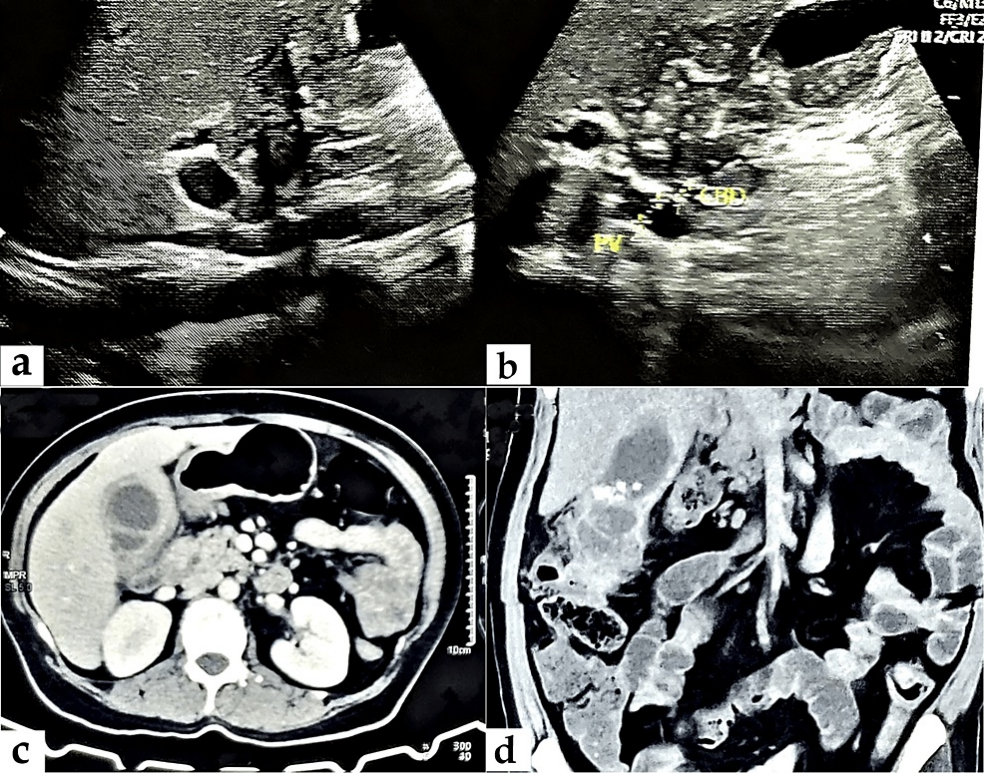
(a) and (b) Gray-scale US images show irregular thickening of the gallbladder wall; (c) axial view of the CECT image; and (d) coronal view of the CECT image showing multiple small calculi, abnormal gallbladder wall thickening, loss of a fat plane with the adjacent liver, and hypoattenuating intramural nodules. US: ultrasound; CECT: contrast-enhanced CT
